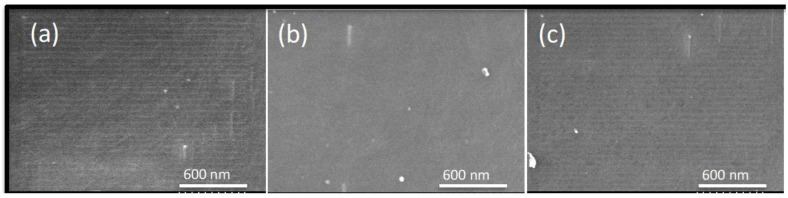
Surface SEM images of dry PIMs with a composition of 30%IL–70%CTA. (a) AlqCl; (b) AlqNO_3_; and (c) AlqSCN.
